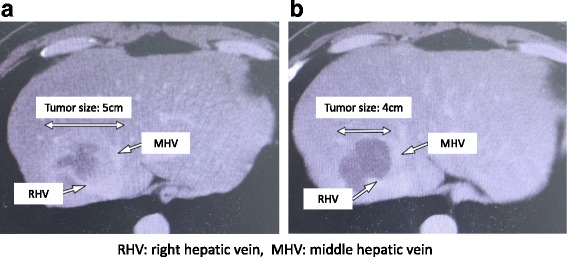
Preoperative computed tomography images of the liver, before (a) and after (b) chemotherapy. *RHV* right hepatic vein, *MHV* middle hepatic vein
